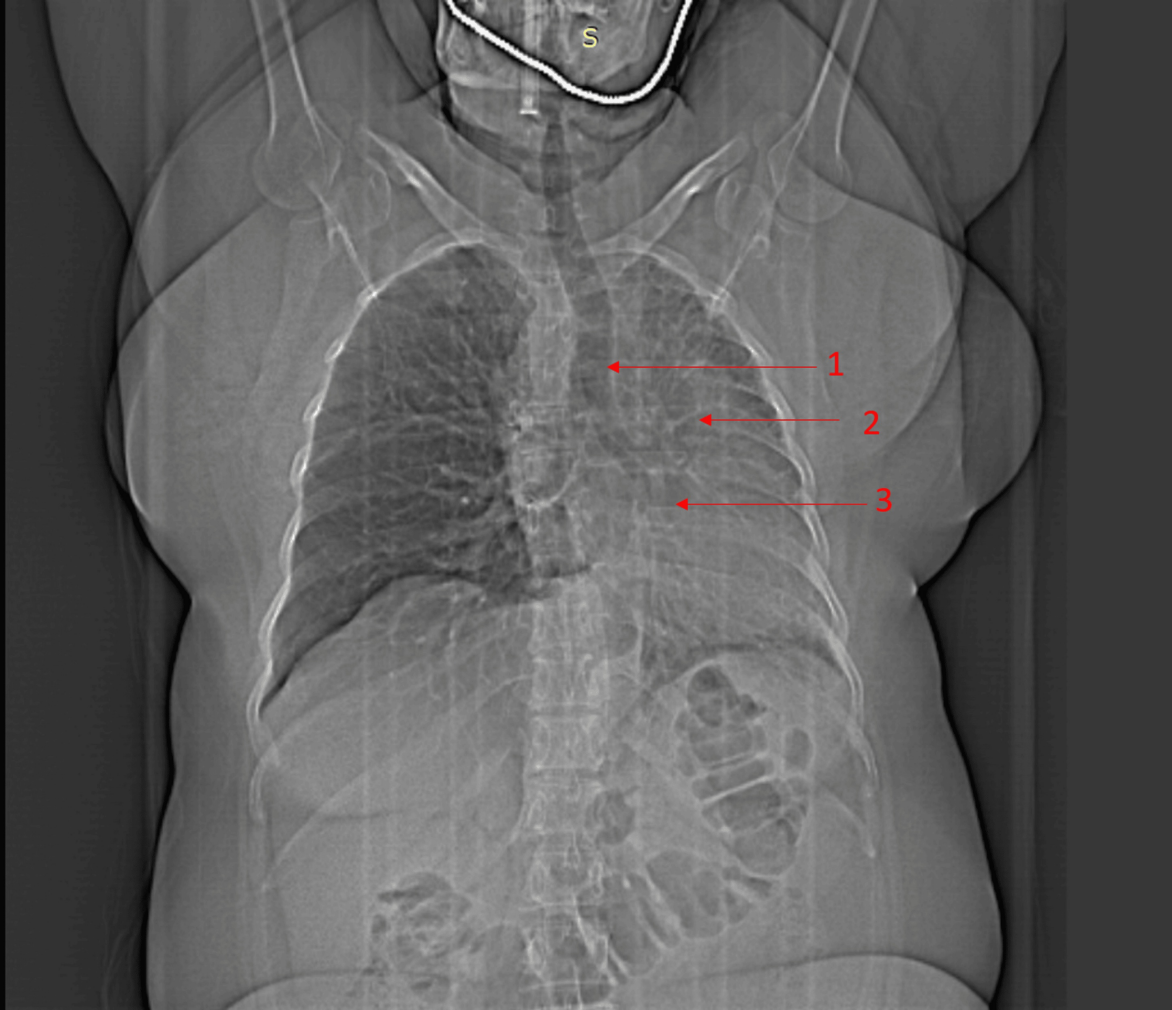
Case 2: X-ray and scout film 1 shows the trachea and mediastinal deviation to the left side. 2 shows the reduced lung volume on the left side. 3 shows the obscured hilar shadow on the left side.
